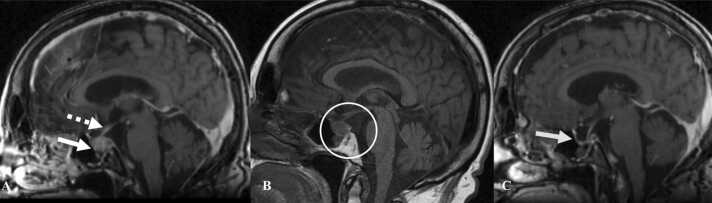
Immune-mediated hypophysitis in a 70-year-old woman with melanoma on Pembrolizumab. A. Contrast-enhanced sagittal MRI image of the brain shows enlargement of the pituitary gland with convex superior margin (arrow) and thickened stalk (dashed white arrow). B. T1W sagittal MRI image of the brain shows absent posterior pituitary bright spot (white circle). C. Contrast-enhanced sagittal MRI image of the brain obtained after Pembrolizumab was stopped and steroid was administered shows significant decrease in the size of pituitary gland with empty sella turcica (arrow).
